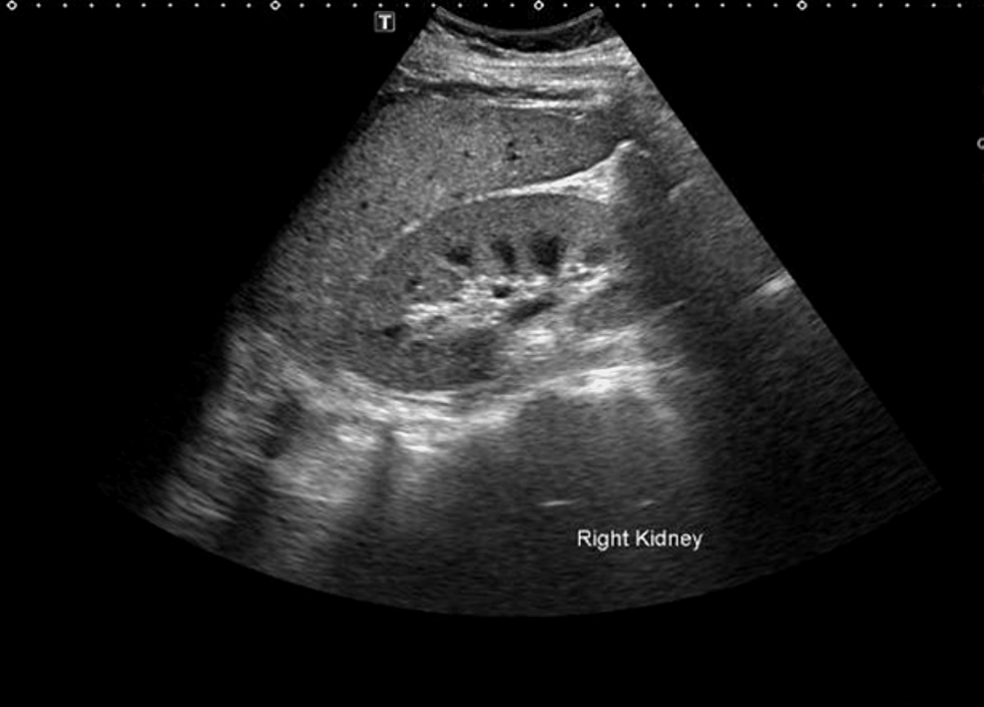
Ultrasound of the right kidney Ultrasound shows an increase in renal cortical echogenicity. Kidney size and cortical thickness are preserved.
